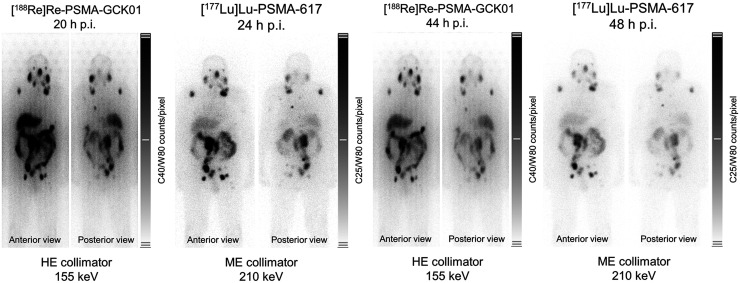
Intraindividual comparison of 3.7 GBq of Lu-PSMA-617 and 3.7 GBq of Re-PSMA-GCK01 at 20–24 and 44–48 h after injection, respectively. HE = high-energy; ME = medium-energy; p.i. = after injection.
